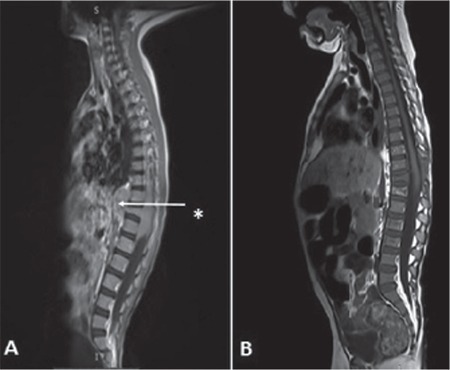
A) Sagittal T1-weighted MRI image showing an epidural, hypointense, craniocaudal mass of 4.5 cm in diameter compressing the spinal cord at the level of D 10-12; B) image of the mass 1 month before completion of acute myeloid leukemia maintenance therapy
